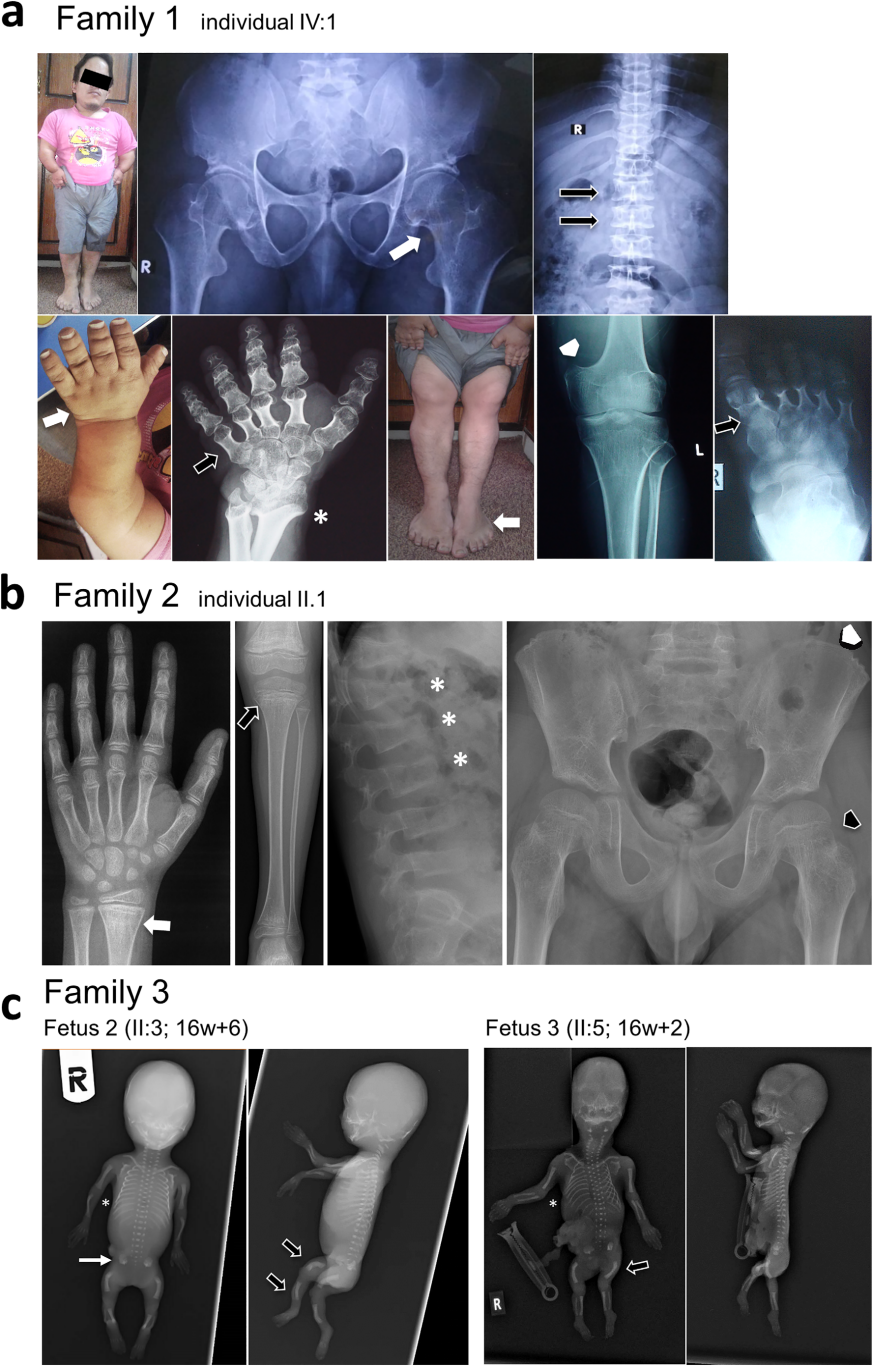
Clinical phenotype in the affected participants. (*A*) Photographs and radiographs of individual IV:1 from family 1. Photograph shows disproportionate short stature with acromesomelic shortening of forelimbs and facial hypoplasia with broad forehead. Hands and feet are short and broad on photographs (lower panel, white arrows) and radiographs show extremely short tubular bones (black arrows) and abnormal distal radius and ulna (white asterisk). Pelvic radiograph shows short and broad femoral necks (upper panel, white arrow). Radiograph of the spine shows platyspondyly (upper panel, black arrows). Knee radiograph indicates wide metaphyses (white arrowhead). (*B*) Radiographs of the affected individual from family 2. Radiograph of the hand shows osteopenia and an irregular mineralization pattern at metaphyses (white arrow). Radiograph of the lower limb shows osteopenia and metaphyseal irregularities (black arrow). Radiographs of the spine indicate significant osteopenia and platyspondyly (white asterisks). Pelvic radiograph shows in addition to generalized osteopenia, mild coxa valga with short and broad femoral necks (black arrowhead) and mild irregularity in the iliac wing (white arrowhead). (*C*) Radiographs of two of the affected fetuses from family 3 (fetus 2 [F22], 16 weeks’ gestation +6; fetus 3, 16 weeks’ gestation +2). Note in both fetuses the small round ilium with trident acetabulum (white arrow) and shortening and bowing of the long tubular bones (black arrows). Thorax appeared slightly narrow (white asterisks).
